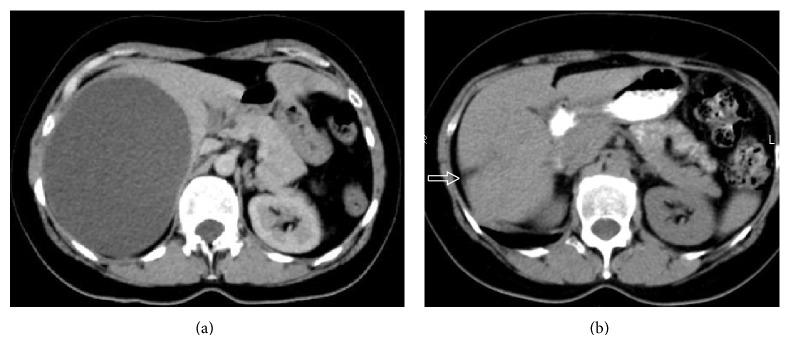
Axial Computed Tomography scans of a 48-year-old Caucasian woman with a large simple cyst located at segment IV of the liver [cyst number 3], before (a) and 6 months after percutaneous sclerotherapy with combination of hypertonic NaCl 15% and bleomycin (b). After treatment [image b], only a simple scar is obvious in the liver parenchyma [arrow].
